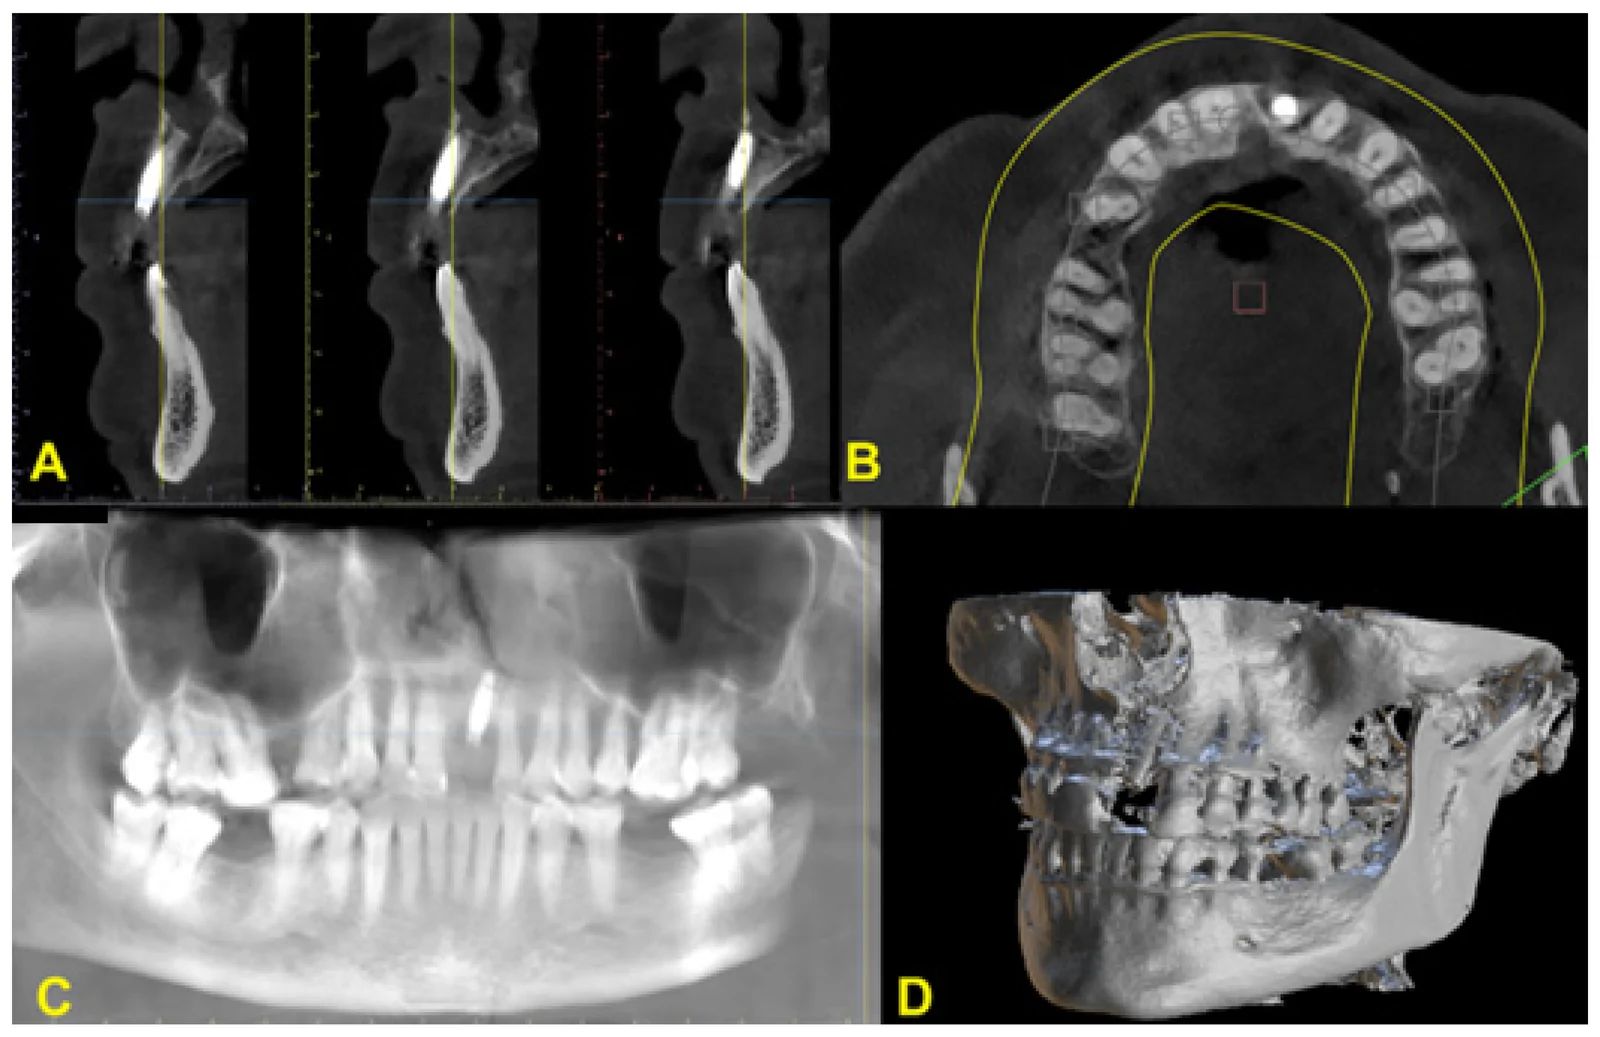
Cone-beam computed tomography of tooth 21 position after first surgery: (A) cross-sectional views; (B) axial view; (C) orthopantomographic view; (D) pseudo-3D reconstruction.
